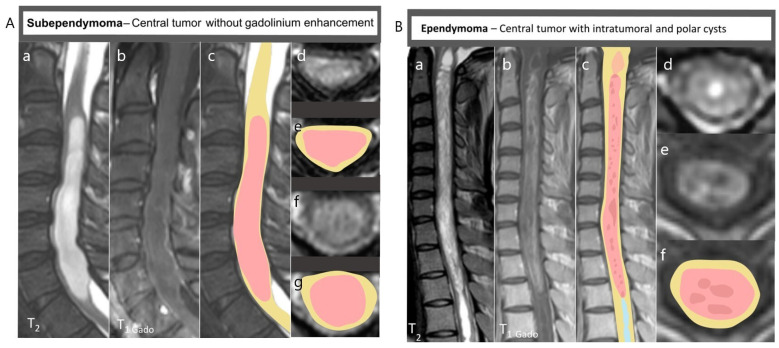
(A) Subependymoma: (a) Sagittal T2-weighted image showing an extensive well-defined hypersignal-T2 mass within the cord; (b) Sagittal T1-contrast-enhanced image showing the absence of gadolinium enhancement; (c) Sagittal pictorial representation; (d,f) Axial T2-weighted image showing the central tumor; (e,g) Axial pictorial representation. (B) Ependymoma: (a) Sagittal T2-weighted image showing an extensive well-defined mass with multiple intumoral cysts and polar cyst; (b) Sagittal T1-contrast-enhanced image showing the gadolinium enhancement of the tumor associated with the polar cyst; (c) Sagittal pictorial representation; (d) Axial T2-weighted image showing a central tumor with an intratumoral cyst; (e) Axial T1-gadolinium enhanced image showing gadolinium enhancement of the tumor without enhancement of the cysts; (f) Axial pictorial representation.
